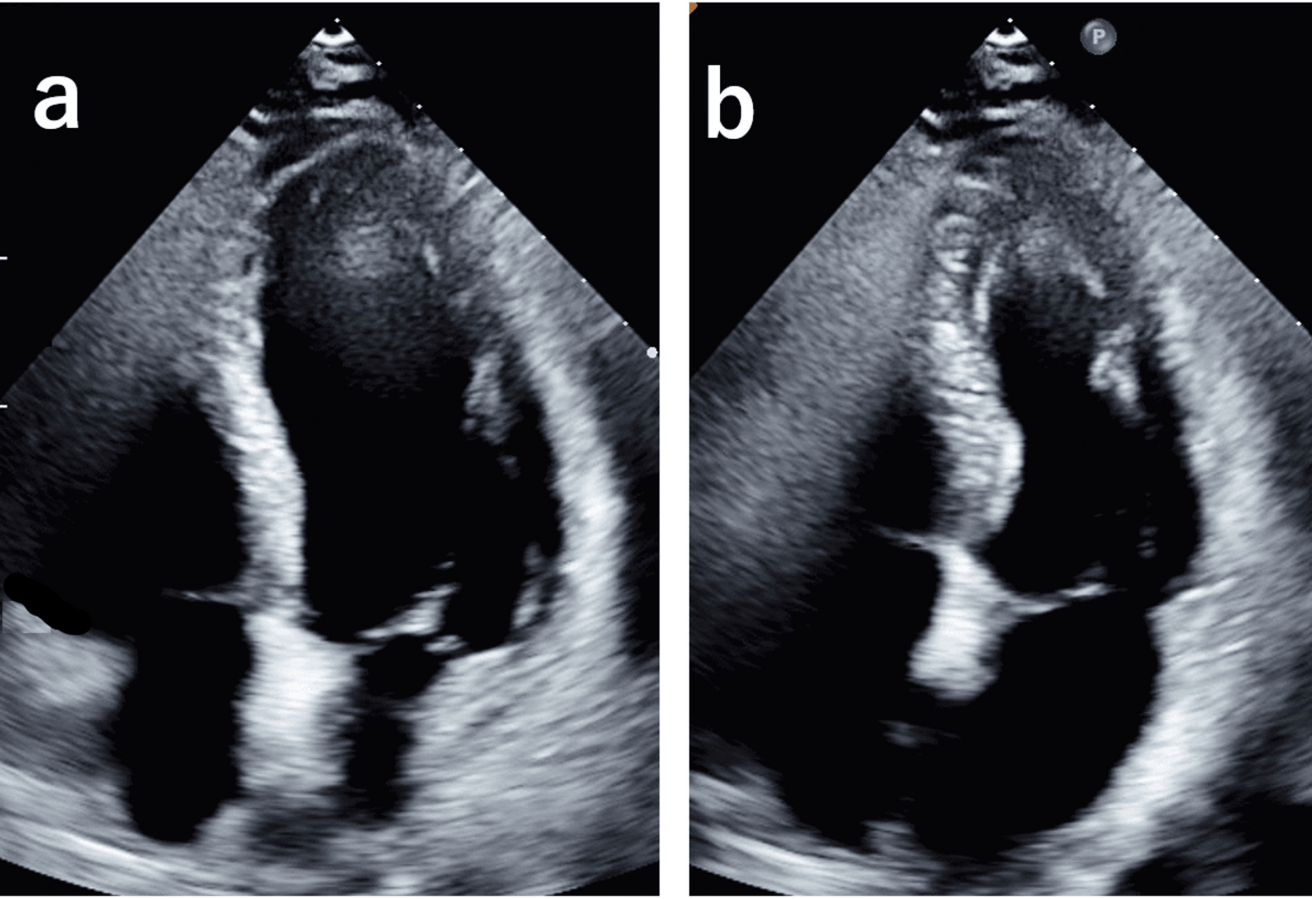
Echocardiography Apical four-chamber view: (a) diastole and (b) systole. Left ventricular ejection fraction: 58%; no significant valvular disease; no left ventricular wall motion abnormalities; no pericardial effusion
